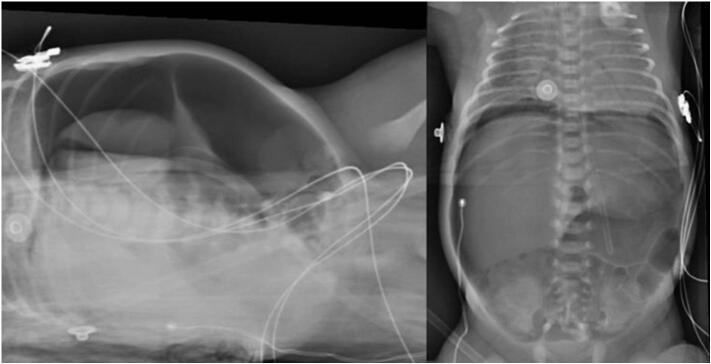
Anteroposterior and Right lateral decubitus abdominal radiograph showing pneumoperitoneum.
